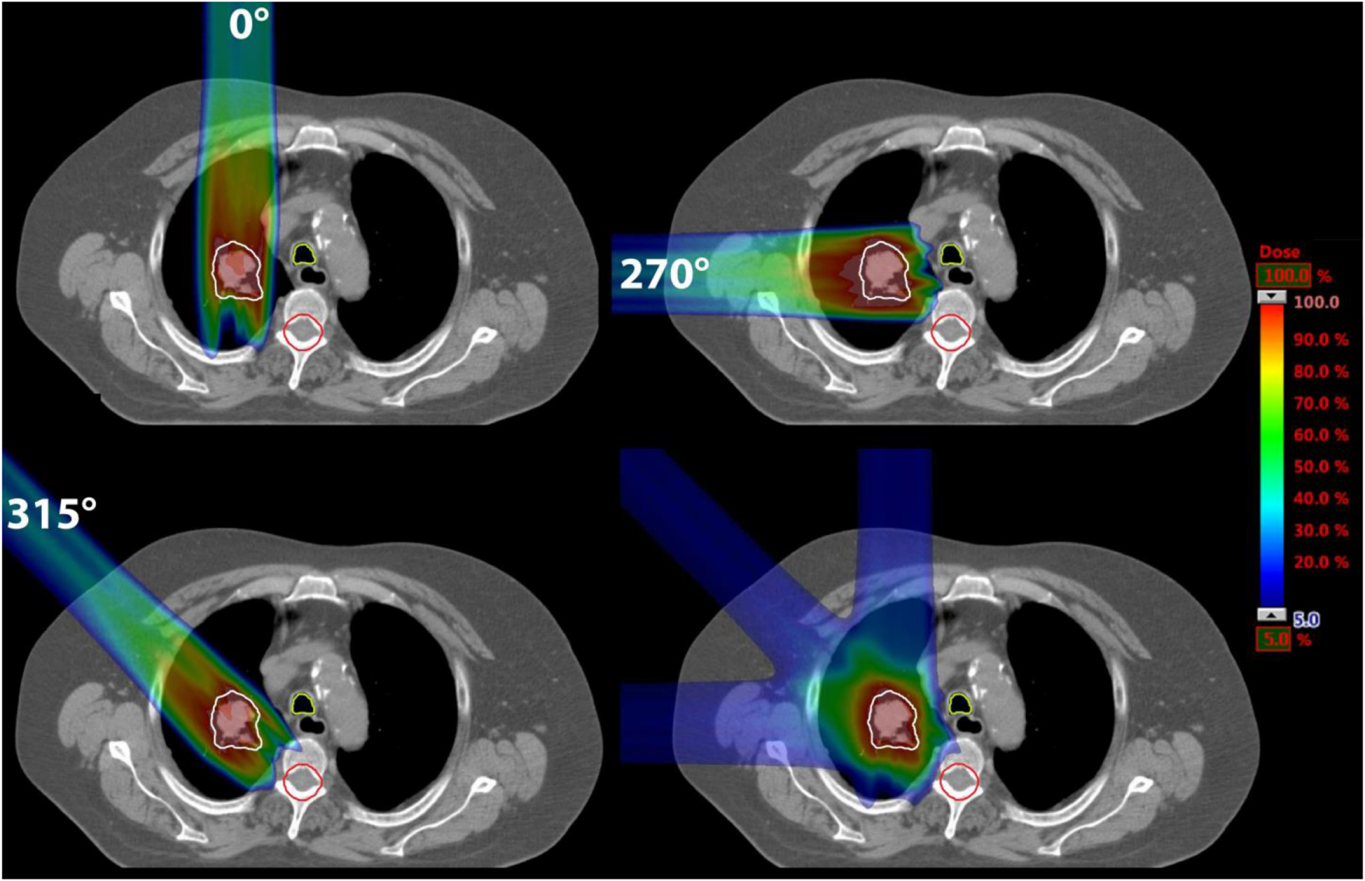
CT slices of one exemplary patient (patient 1): for the beam directions 0°, 270° and 315° plans were optimized individually consisting of one single field. On the bottom right the sum of these plans is shown. The CTV is marked in white, trachea in light green and spinal cord in red. On the right a color bar is given indicating the relative dose
